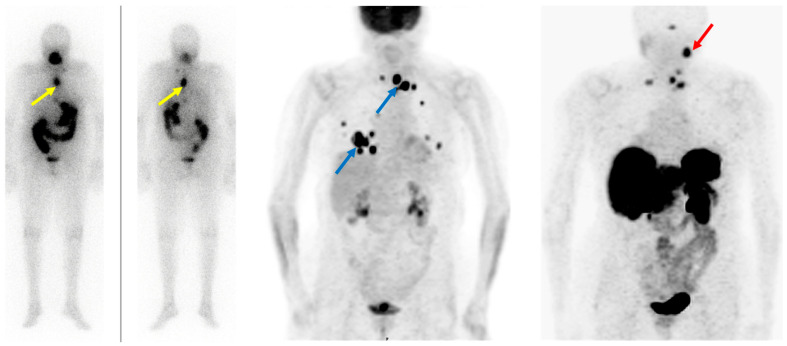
Radioiodine-refractory metastatic differentiated thyroid cancer with positive lesions on [^18^F]FDG PET and [^68^Ga]Ga-DOTANOC PET. Legend: Eighty-four-year-old patient with advanced follicular thyroid cancer and (new) pulmonary and lymph node metastases, after 17 GBq ^131^I, Tg: 8660 ng/mL. (Left): diagnostic ^131^I imaging showing radioiodine-avid lymph node metastases (yellow arrows) in the chest. (Middle): [^18^F]FDG PET depicts discordance with RAI-imaging lymph node metastases in the upper mediastinum and lung metastases (blue arrows). (Right): [^68^Ga]Ga-DOTANOC PET shows SSTR-expression in some but not all lymph node metastases in the upper mediastinum; additionally, visualization of a lesion in the left neck not seen with [^18^F]FDG and ^131^I (red arrow). However, no SSTR expression in the lung metastases was observed.
